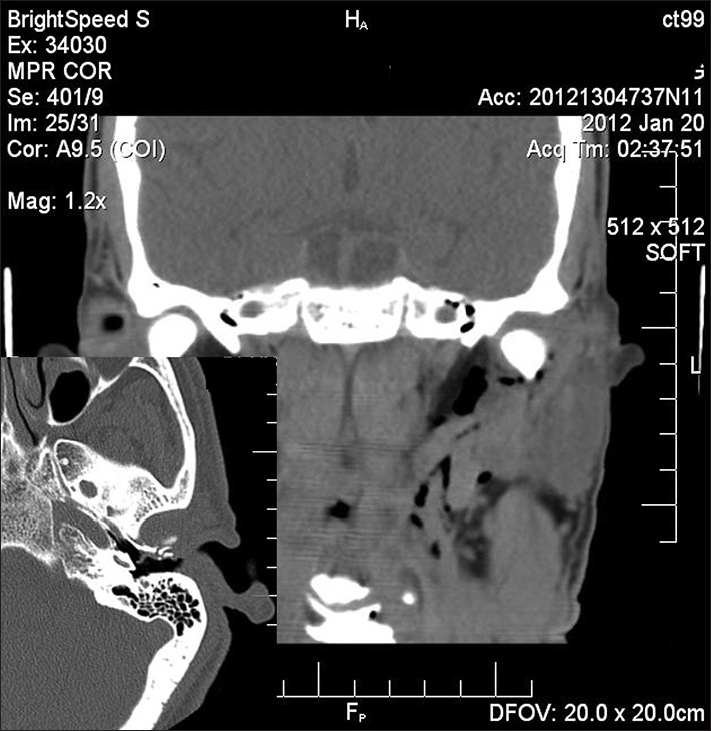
Coronal and axial CT images. External auditory canal anterior wall fracture and consequent emphysema of the ipsilateral parapharyngeal space.
